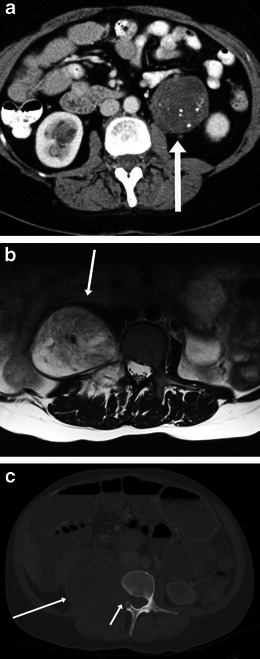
Imaging of neurofibroma. a CT scan in a 64-year-old woman showed an incidental well defined hypodense mass with speck of calcification (*arrow*) in the left retroperitoneum. b, c A 45-year-old woman with lower abdomen/thigh pain. Axial T2 MRI (b) showing a heterogeneous, high signal lobulated mass at L3/L4 level (*arrow*). The right L3 nerve root was not separately identified. CT scan (bone window settings) at the same level (c) shows the mass (*arrow*) exiting from the widened neural foramina without causing bony destruction (*small arrow*)
